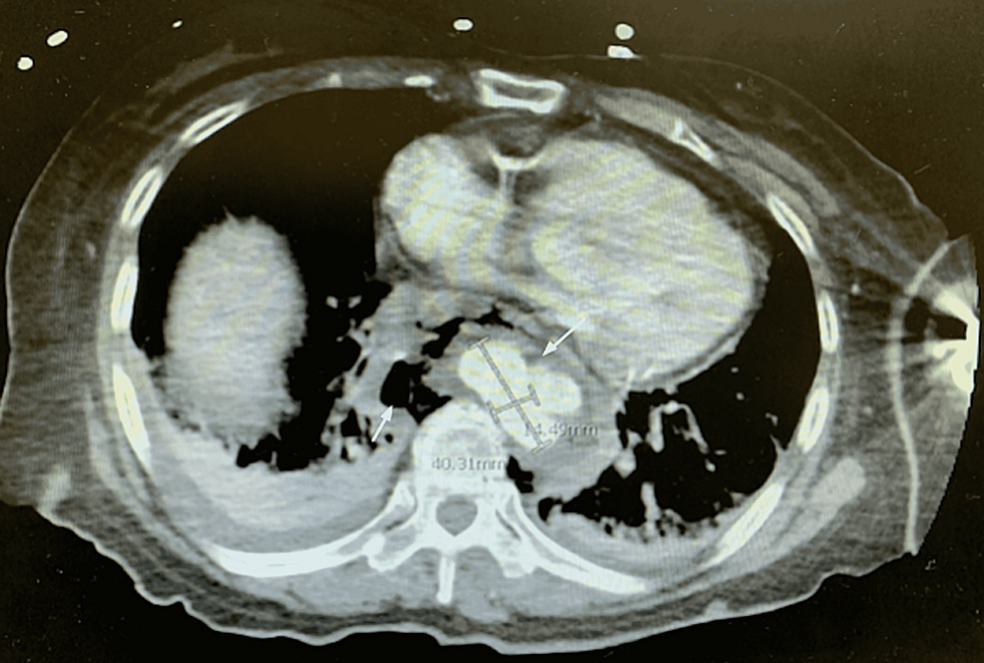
CT chest with contrast demonstrating a pseudoaneurysm of the aorta (measurements) and air-fluid levels in surrounding tissues (arrows).
